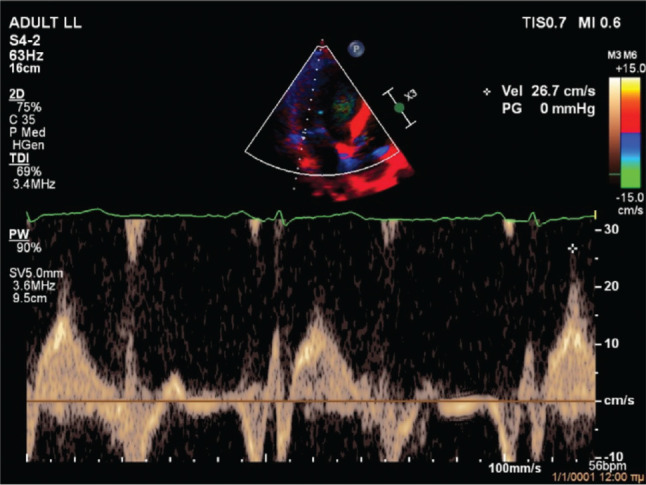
Case 2 tissue Doppler imaging showing pickelhaube sign (peak systolic lateral mitral annulus velocity of ≥16 cm/s resembling the “pickelhaube,” a spiked helmet).
